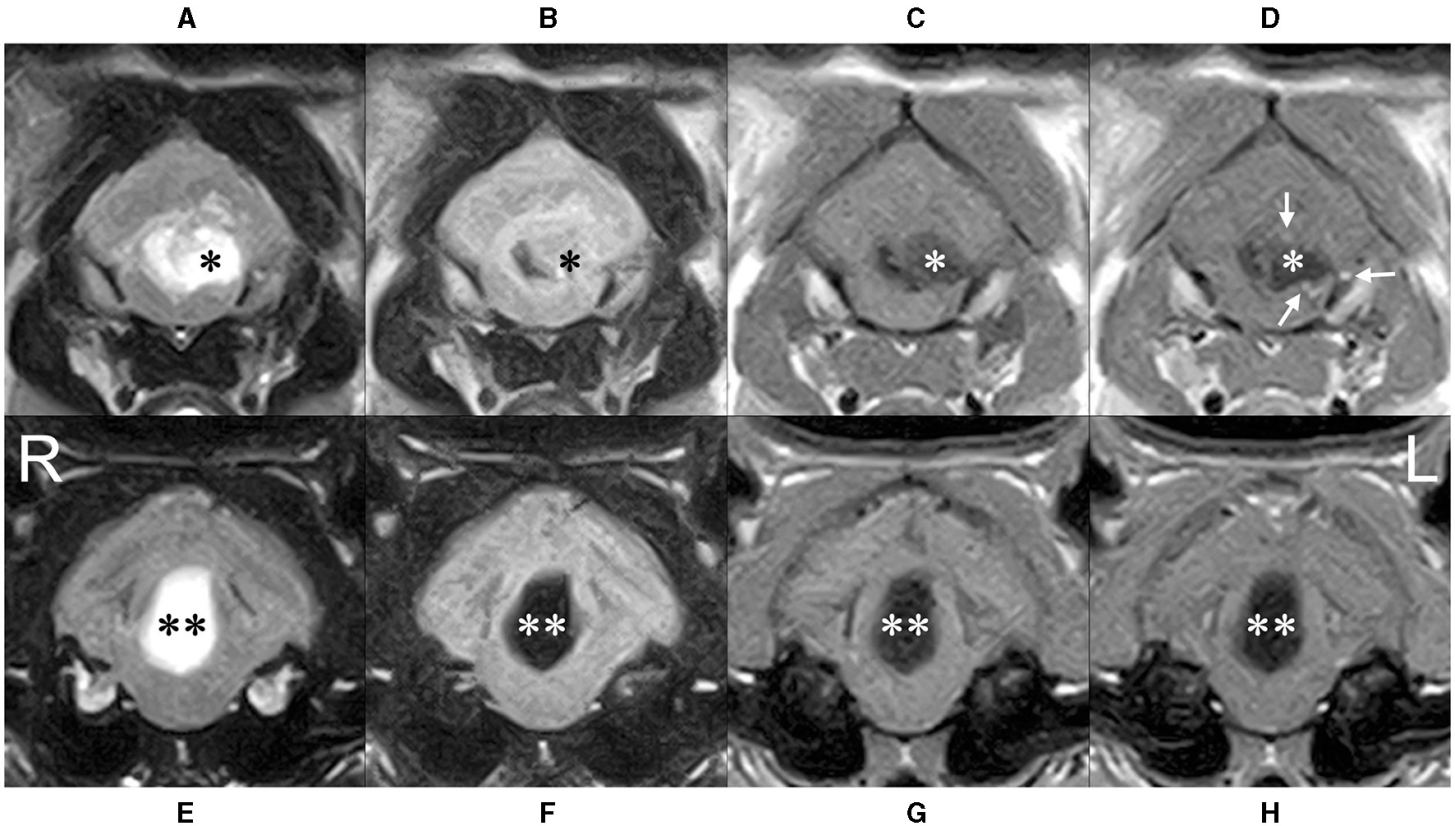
Transverse MRI at caudal (A–D) and rostral (E–H) level of the cerebellum. The cyst (*) severely compressed the fourth ventricle to the right, the medulla ventrally, and the cerebellum dorsally. High intensity on T2-weighted (A) and FLAIR (B) imaging, low intensity on T1-weighted imaging (C) (higher than cerebrospinal fluid) was observed, and contrast-enhanced T1-weighted imaging (D) showed a ring-shaped enhancement of the limbus (white arrows). The rostral cystic lesion () showed high intensity on T2-wighted imaging (E), lower intensity than that of cerebrospinal fluid on FLAIR imaging (F) and low intensity on T1-wighted imaging (G) without any contrast enhancement (H).
